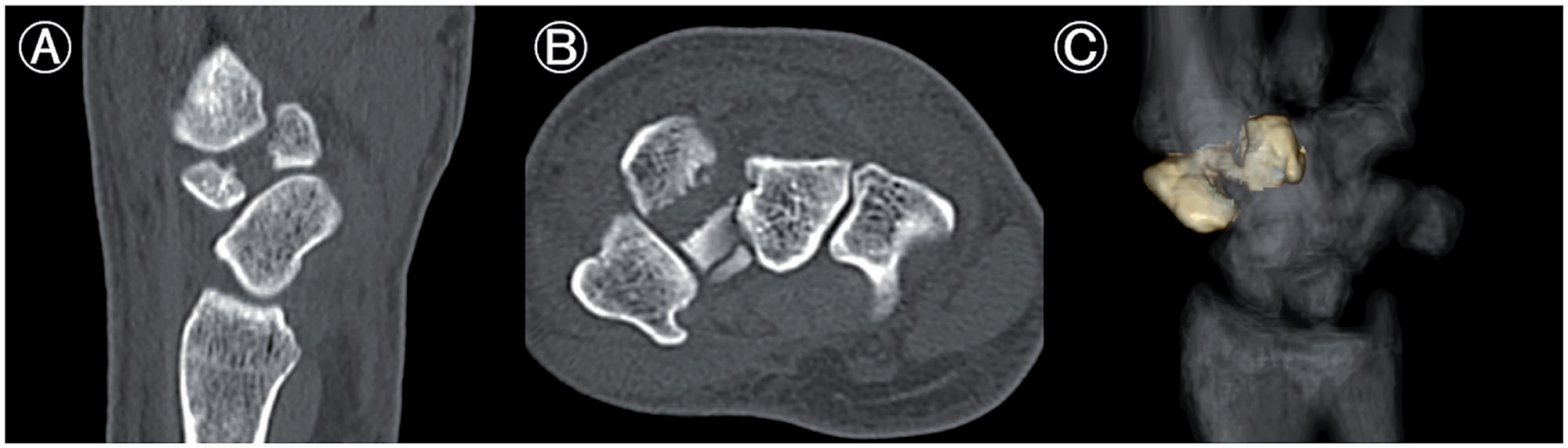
Computed tomography (CT) image of an isolated left trapezoid coronal shearing fracture in a 40-year-old male. (A) The preoperative sagittal CT image shows a coronal shear fracture at the center of the left trapezoid with a gap of 6 mm. The base of the second metacarpal bone is interposed between the fracture fragments. (B) The axial CT image shows that the palmar fragment of the trapezoid is in its original position, but the dorsal fragment is displaced dorsally. (C) On the three-dimensional CT, only the trapezoid is highlighted. A large gap and dorsal displacement of the dorsal fragment is confirmed anatomically and three-dimensionally. No abnormal positioning of the trapezoid or the other carpal bones is observed.
